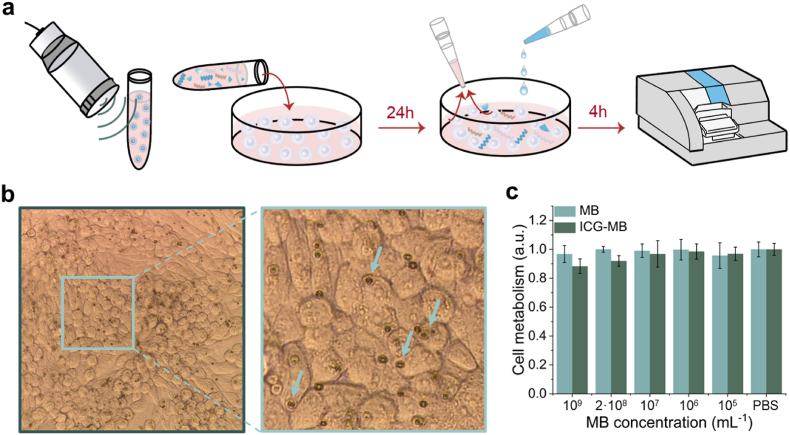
Cytotoxic effects of indocyanine green (ICG)-coated microbubbles. (a) Steps of the cell viability assay. From left to right – 1) microbubble disruption with ultrashort ultrasound pulses, 2) feeding of the Chinese hamster ovary (CHO) cell culture with microbubble debris, 3) addition of alamarBlue solution to the cell culture and subsequent sampling, 4) measurement of fluorescent signals. (b) Bright field optical image of the cells prior to addition of alamarBlue solution. Internalized particles are indicated with arrows. (c) Results of the alamarBlue cell viability assay for cells incubated with different concentrations of ICG-coated microbubbles. (For interpretation of the references to color in this figure legend, the reader is referred to the Web version of this article.)
